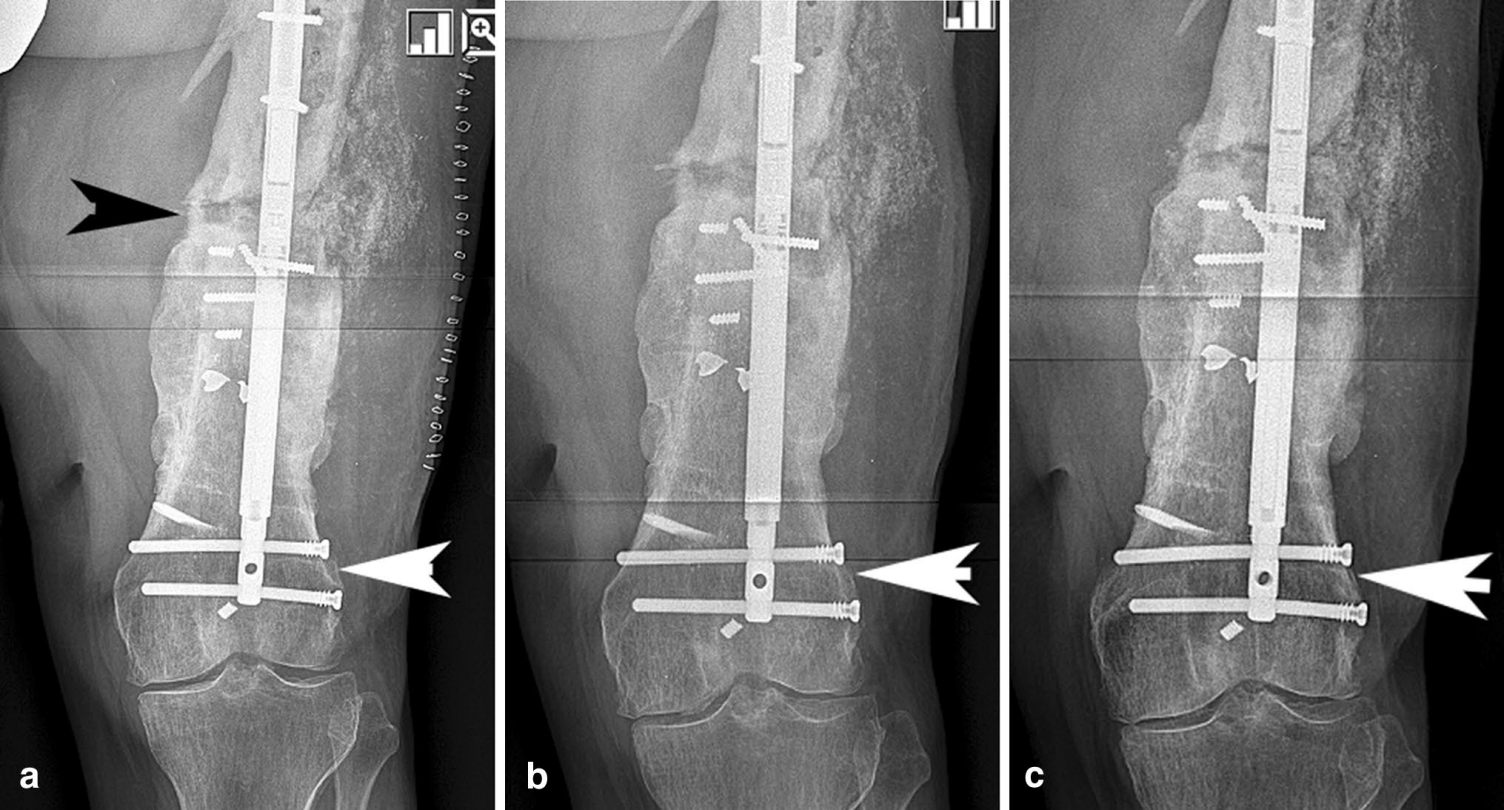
a This AP X-ray shows a patient (case #3) with bending of the distal locking bolts (white arrow) indicating strong compression at the nonunion site (black arrow). b The same patient underwent radiographic examination one month later with visible loss of bending of the screws (arrow) indicating a loss of compressive load at the bone ends. Additional compression of 2 mm per day for 2 days was applied after this visit. c The same patient had X-rays taken one month later demonstrating screw bending (arrow), evidence that compression was present at the nonunion site
